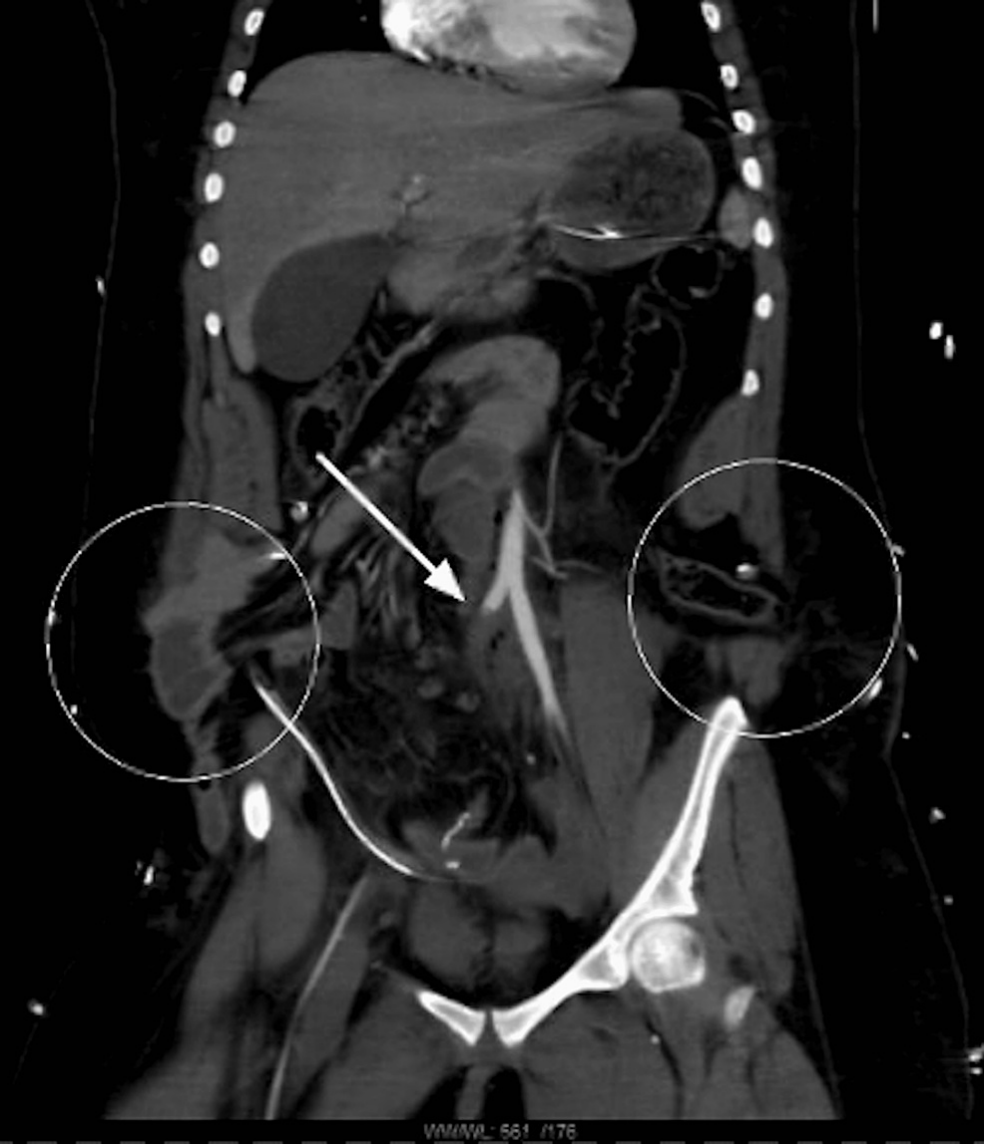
CT scan of the patient’s postoperative abdomen depicting iliac artery injury (arrow), with circles highlighting the ostomy and a hernia left behind. CT, computed tomography
